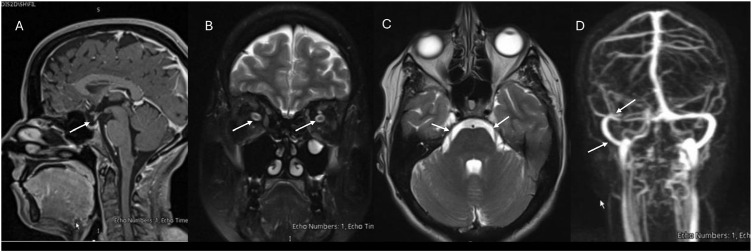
(A) Magnetic resonance imaging (MRI), sagittal view, T1 sequence with intravenous gadolinium showing partial empty sella turcica (arrow). (B) T2 sequence, coronal view showing bilateral optic nerve sheath swelling (arrows). (C) T2 sequence, axial view showing dilated Meckel’s caves (arrows). (D) MRI venogram showing hypoplastic right transverse sinus and mild narrowing in right sigmoid sinus (arrows).
